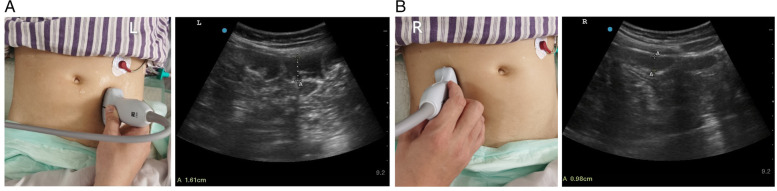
Left semicolon ultrasound and Right colonic ultrasound. A: The Sonosite M-Tube to was used for monitoring, with the 2–5 MHz convex array probe. The ultrasound probe was placed in the front line of the left axilla, and the direction of the probe was parallel to the longitudinal axis of the body, then obtained a clear image of the descending colon on the left. Then we measured the left descending colon diameter. B: The Sonosite M-Tube to was used for monitoring, with the 2–5 MHz convex array probe. The ultrasound probe was placed in the front line of the right axilla, and the direction of the probe was parallel to the longitudinal axis of the body, then obtained a clear image of the right ascending colon. Then we measured the inner diameter of the right ascending colon
